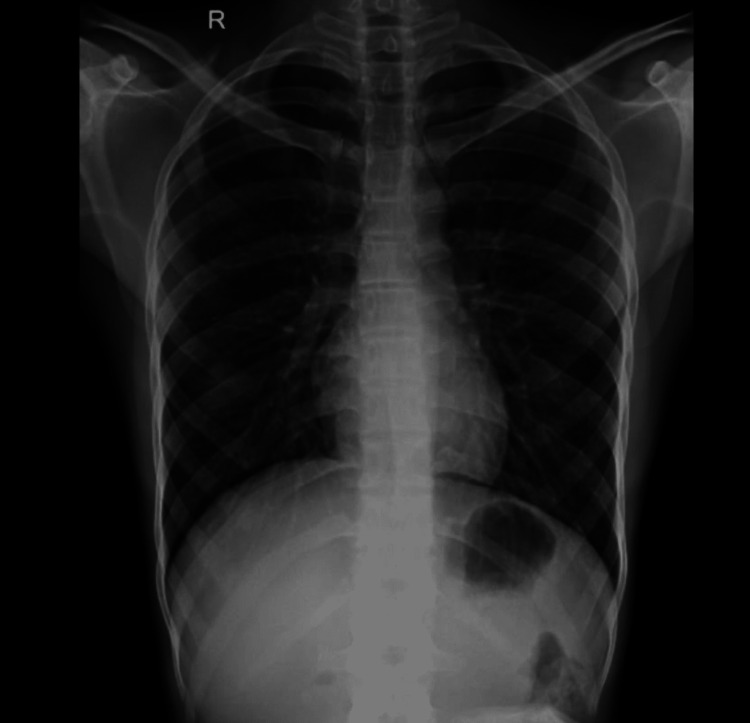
X-ray of the thorax (posteroanterior) view showing a clear chest.
